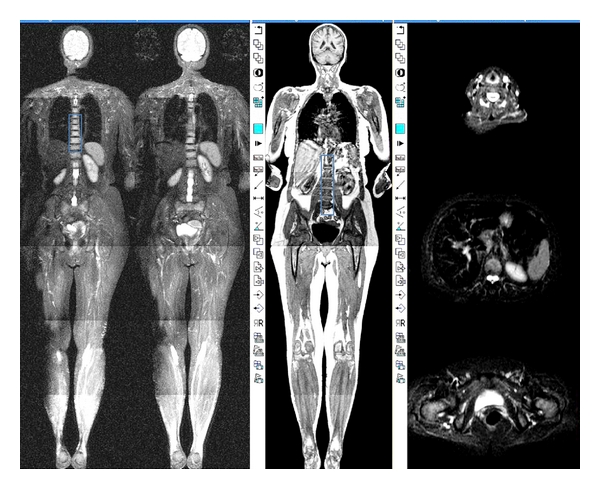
Whole-body MRI coronal and selective axial STIR sequence. Left image: coronal STIR sequence demonstrating T2 bright myelomatous disease throughout the thoracic spine (rectangle). Centre image: coronal T1-weighted sequence demonstrating low signal marrow throughout the lumbar spine due the myelomatous infiltration (rectangle). Right image: 3 axial MRI images at the level of the vocal cords, lumbar spine, and ischium, T1-weighted sequence following administration of contrast.
